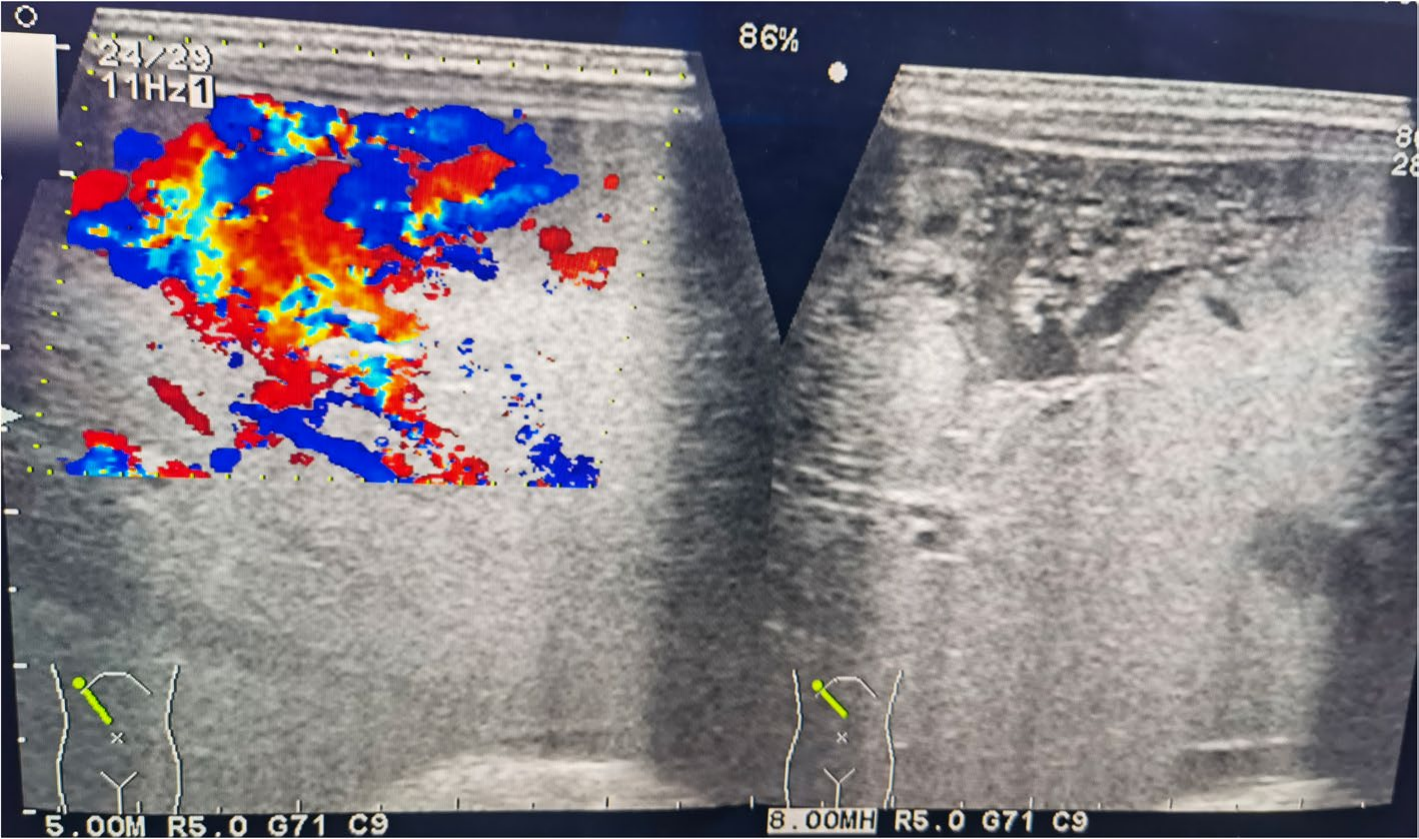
Abdominal ultrasound showed cavernous transformation in the liver with the intralesional massive turbulent vascular flow
